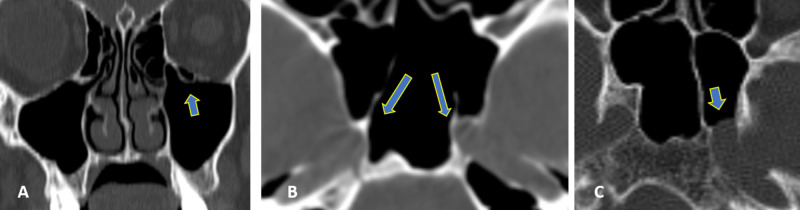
CT images with variations (arrows) (A) Left Haller cells, (B) sphenoid sinus septa attached to bilateral carotid artery canals, and (C) left carotid artery dehiscence.
